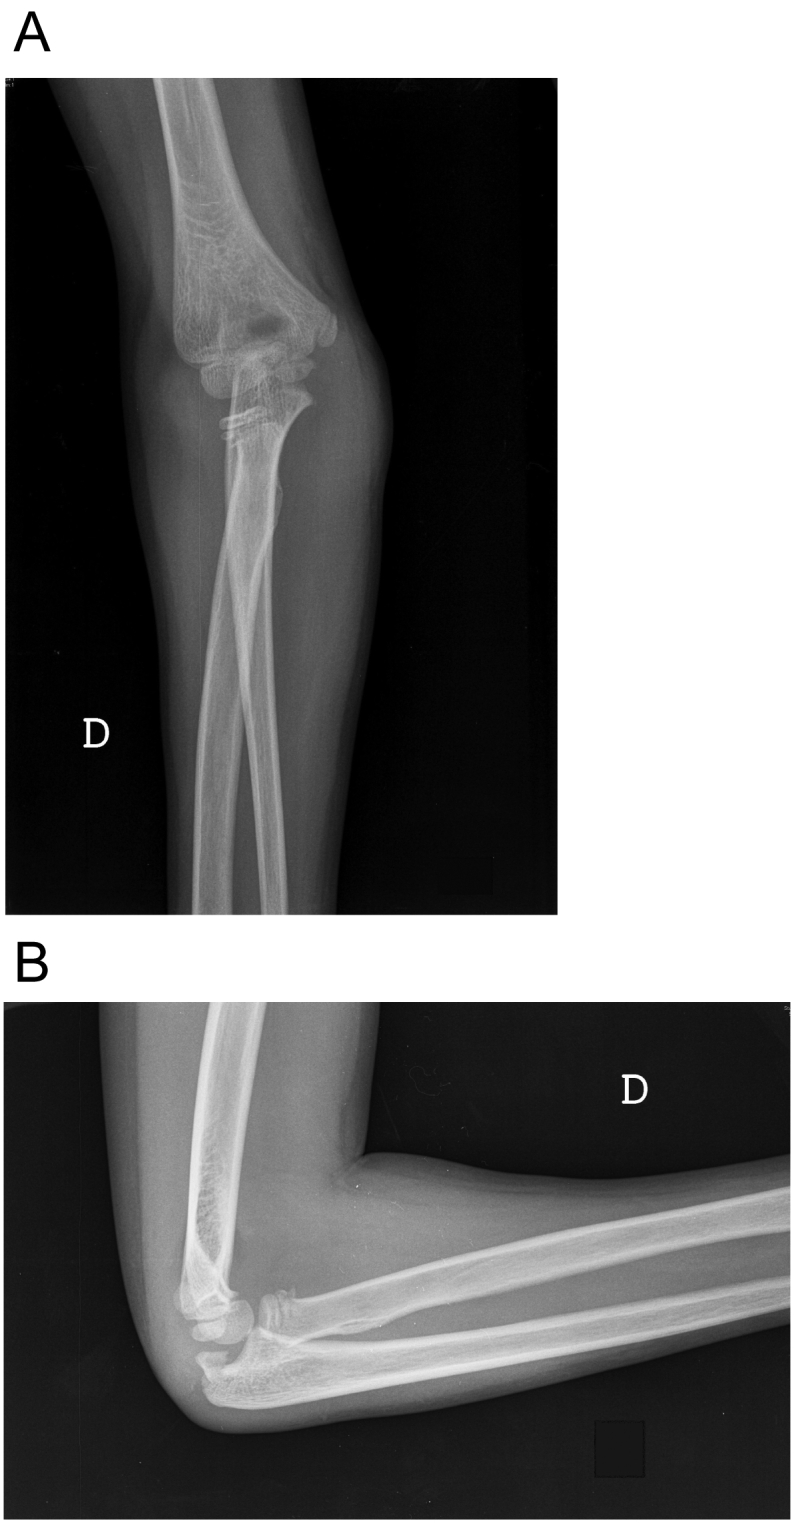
a–b: Standard elbow X-ray showed acute trans-olecranon fracture dislocation of the elbow associated with a radial head fracture.
